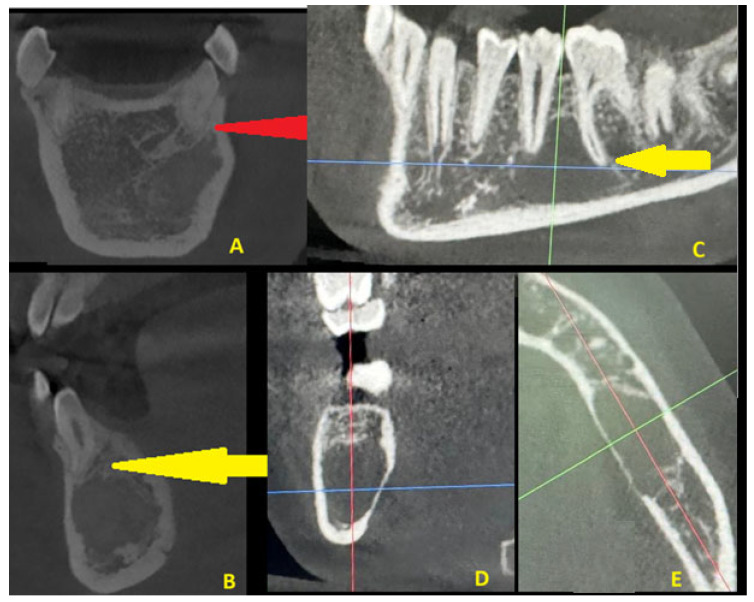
A TBC is an unusual, non-neoplastic, accidentally found pseudocystic cavity, common in the posterior mandible, which is asymptomatic, without any clinical relevance, and slow-growing (C–E). It is mostly found in children and young adults, and sometimes its occurrence might be related to minor trauma. In both cases, trauma or other injuries to the mandible were not confirmed. The lesion itself is an empty bone cavity, without any lining, without calcifications, and it commonly surrounds teeth roots or embeds them within the lesion (C–E). Clinically, teeth remain vital, and the neuro-vascular bundle is intact (yellow arrow) in both COD and TBCs. Normally, COD lesions (A,B) are associated with teeth roots or apices, similar to a TBC; however, sometimes they can be found far from any tooth-bearing structures, which might cause misdiagnosis (red arrow). A radiological comparison in CBCT between COD and a TBC is presented in Figure 5. In a TBC, a radiolucent unilocular lesion has mostly scalloped margins and is either empty or filled with blood or some atypical fluid-like substance. Because both lesions can look like cystic-like lesions, and some lesions of both odontogenic and non-odontogenic origin can look exactly the same, revision surgery with biopsy should be used when radiological appearance and the patient’s surgical anamnesis are not sufficient for any final diagnosis [9,10]. Sometimes, a comparison with future radiographs and a wait-and-see approach is a solution, but each case requires some individual treatment. A study by Park et al. highlights that CBCT is the most accurate diagnostic tool to distinguish COD from other pathologies found in the tooth-bearing regions of the jaws, especially other cyst lesions [5]. On the other hand, additional diagnostics like SPECT (single-photon emission tomography) with blood evaluation for calcium–phosphorus markers are also needed in some cases of endocrine-related jaw bone lesions [1,2,3,4,5,6]. The red, blue, and green axes (C–E) represent the field of radiological visualization of the TBC. To summarize, improved diagnostics with CBCT is quite important to underline the scope of the lesion and the possible necessity for a biopsy. Secondly, any lesions without any calcifications, septa formation, or bone structures within the cystic cavity should be differentiated between a unicystic ameloblastoma, a traumatic bone cyst, early stages of cemento-osseous dysplasia formation, or similar cystic lesions, as all of the mentioned conditions look very similar in CBCT. When a similar radiological appearance is found, revision surgery followed by a biopsy is the most advised diagnostic step to minimize any risk of false diagnosis.
